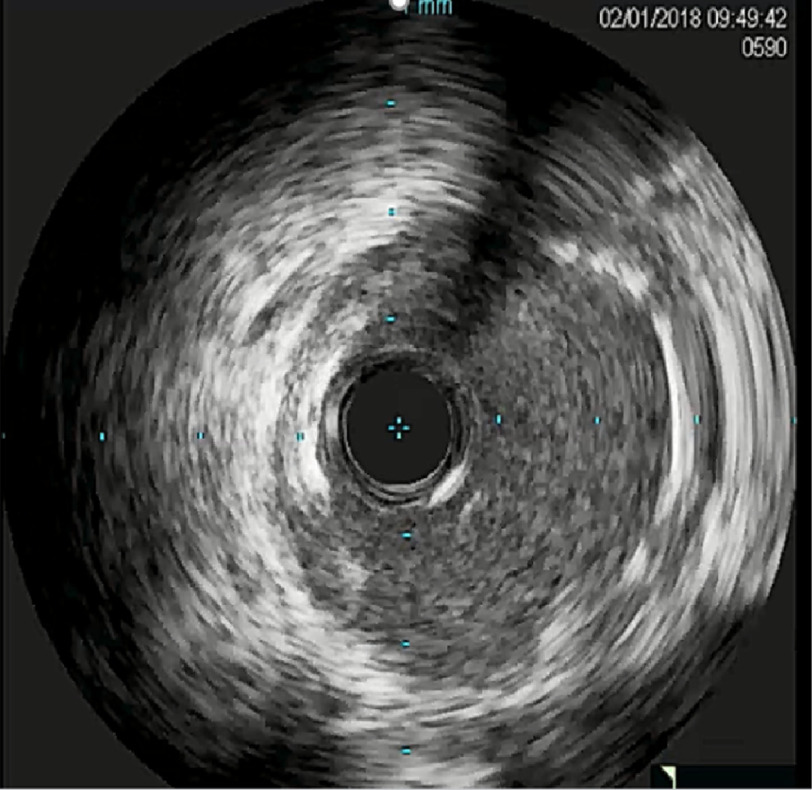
Intra-vascular ultrasound (IVUS) images obtained following stent placement, demonstrating incomplete stent apposition (ISA).
